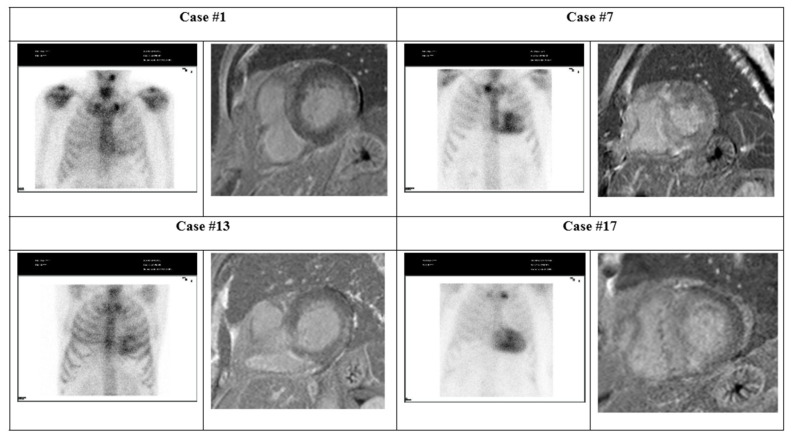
[Cardiac Imaging Evaluation] Patients underwent scintigraphy with 99m Tc-DPD (left) before surgery. Late gadolinium enhancement discernible from cardiac magnetic resonance imaging is evident in the images on the right.
